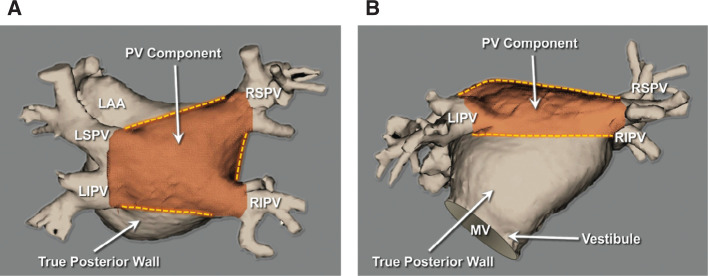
The PV component. A: A cardiac computed tomography image showing a posterior projection of the LAPW, including the PVs and the tissue that lies between them—the so-called PV component. B: On the other hand, the true LAPW spans from the inferior borders of the inferior PVs to the superior margin of the vestibule, the region surrounding the mitral valve orifice. As such, the PV component in essence represents more the dome of the LA than the PW. LAA: left atrial appendage; LIPV: left inferior pulmonary vein; LSPV: left superior pulmonary vein; MV: mitral valve; PV, pulmonary vein; RIPV: right inferior pulmonary vein; RSPV: right superior pulmonary vein.
